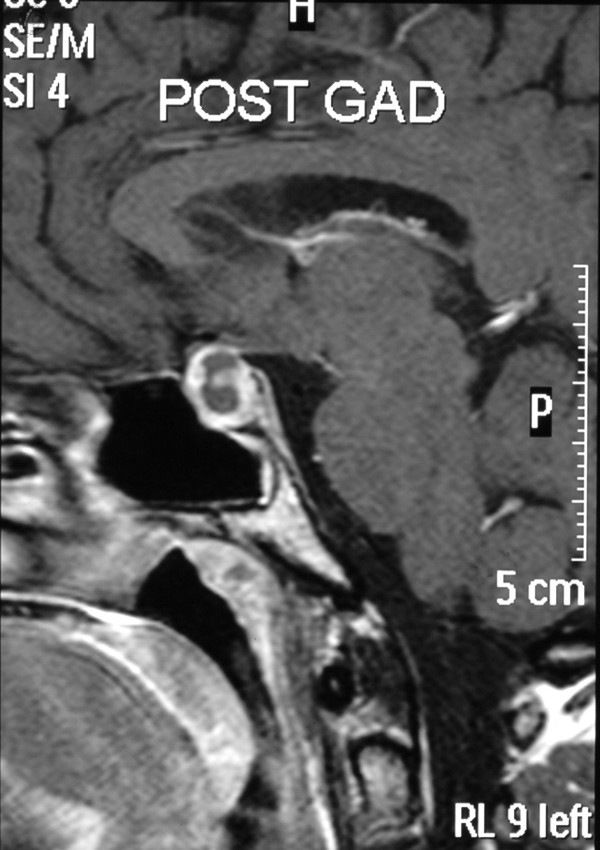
Magnetic resonance T1-weighted contrast-enhanced imaging; sagittal view.
